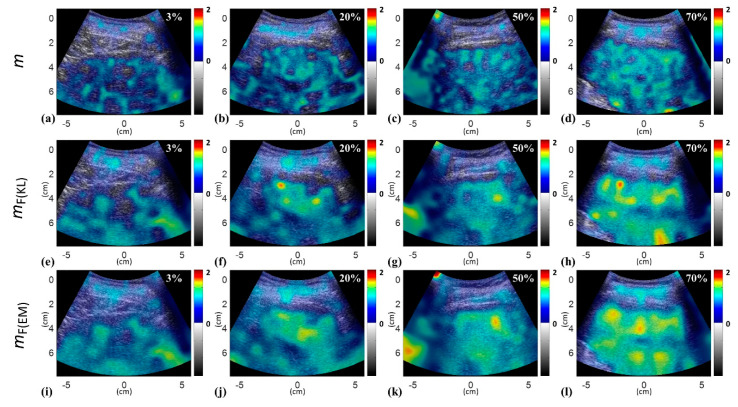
Ultrasound parametric imaging constructed using the *m* (a–d), *m*_F(KL)_ (e–h), and *m*_F(EM)_ (i–l) parameters for different grades of hepatic steatosis. The formation of hepatic steatosis strengthened the image brightness in the Nakagami and the proposed DND imaging, representing increases in the parameters *m*, *m*_F(KL)_, and *m*_F(EM)_.
